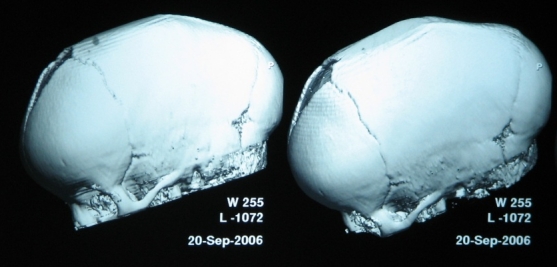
Preoperatory 3D CT in scaphocephaly
